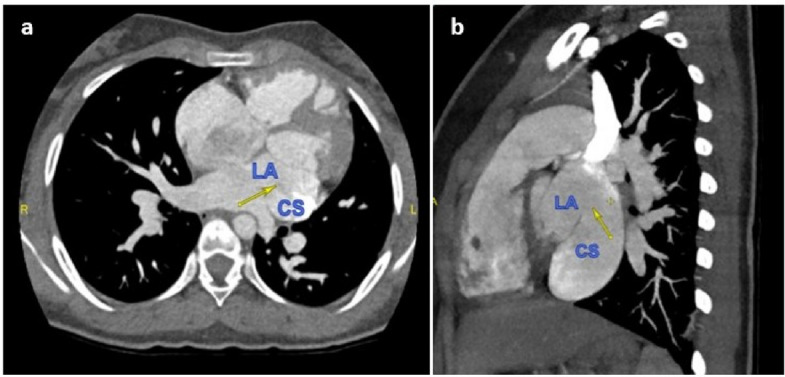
Cardiac computed tomography (A) axial and (B) oblique sagittal views demonstrating dilated coronary sinus (CS) and defect in the posterior wall of left atrium (LA) communicating with CS (arrows)
